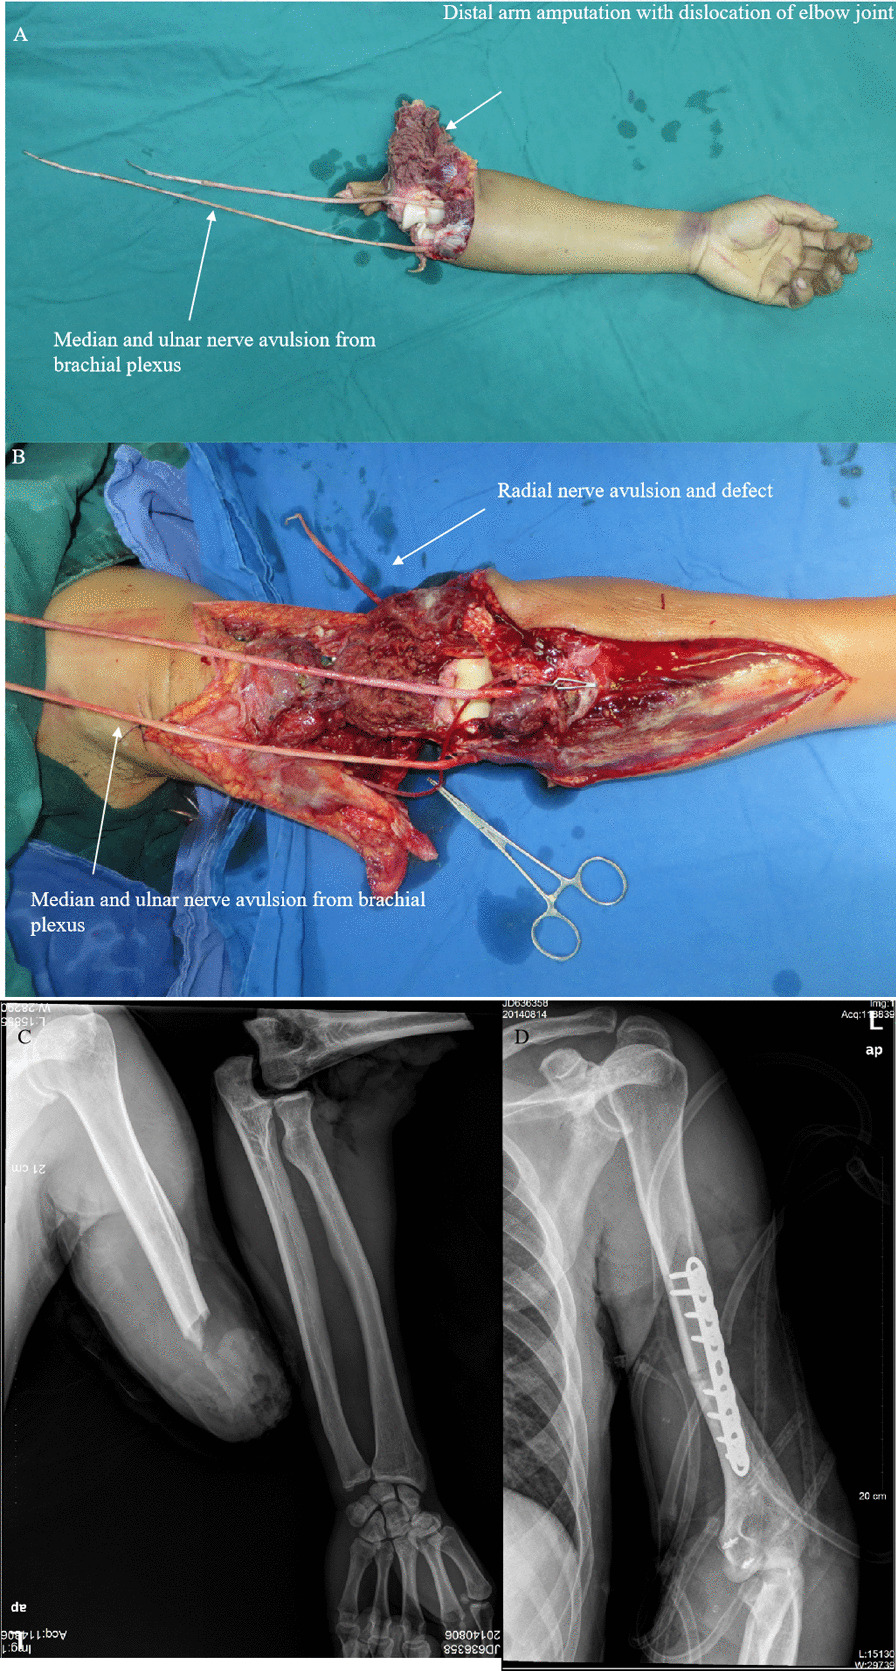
A patient (case 1) undergoing M-(M + R) repair (Part 1). A The left upper arm was severed, which was accompanied by dislocation of the elbow joint and avulsion of the three nerves with defects. B Temporary blood circulation was provided to shorten the warm ischaemia time, elbow joint reduction was performed, and the ligament was repaired. C Preoperative X-ray showed fractures in the distal humerus segment and dislocation of the elbow joint. D Postoperative X-ray showed internal fixation of the humeral fractures, reduction of the elbow joint, and implantation of anchor nails to repair the ligament
